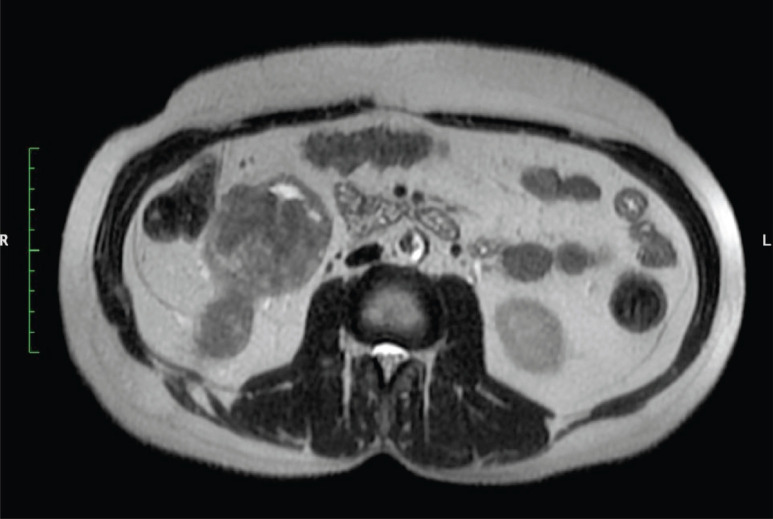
MRI abdomen axial T2 image reveal right lower pole renal tumour with tract of PCNL.
